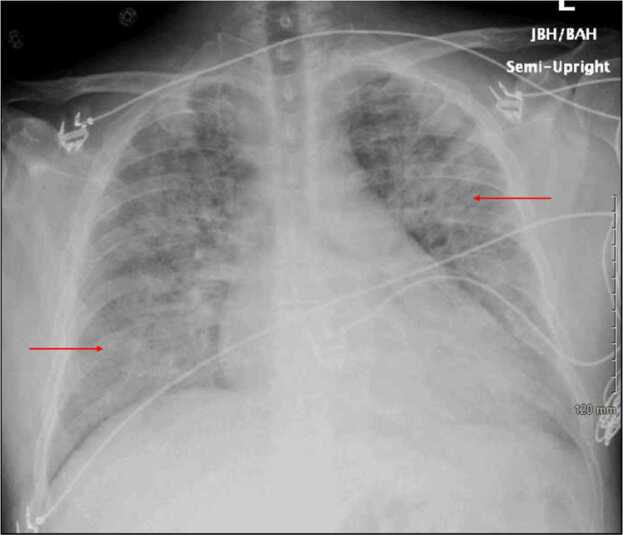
Chest X-ray portable revealed bilateral widespread patchy areas of airspace Opacification (Red arrows.
